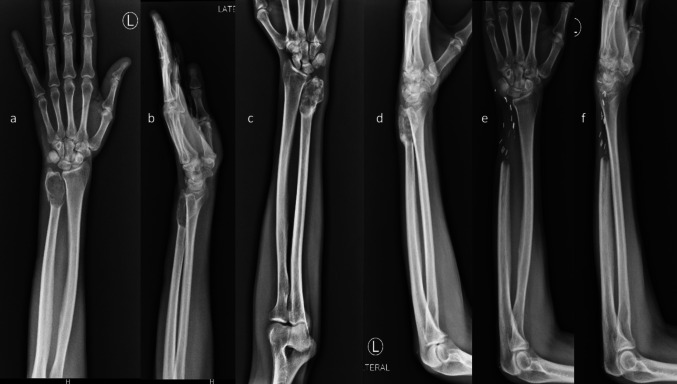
X-ray images a, b showing pathological fracture of ulna on initial presentation, c, d fracture union on denosumab adjuvant and pop slab support, and e, f 1-year follow-up X-ray
